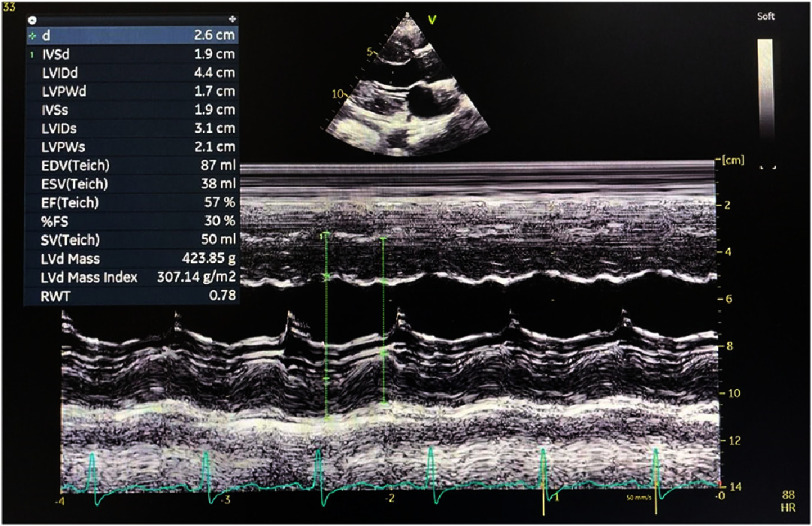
M-mode echocardiography. Intraventricular septal diameter was 1.9 cm.
